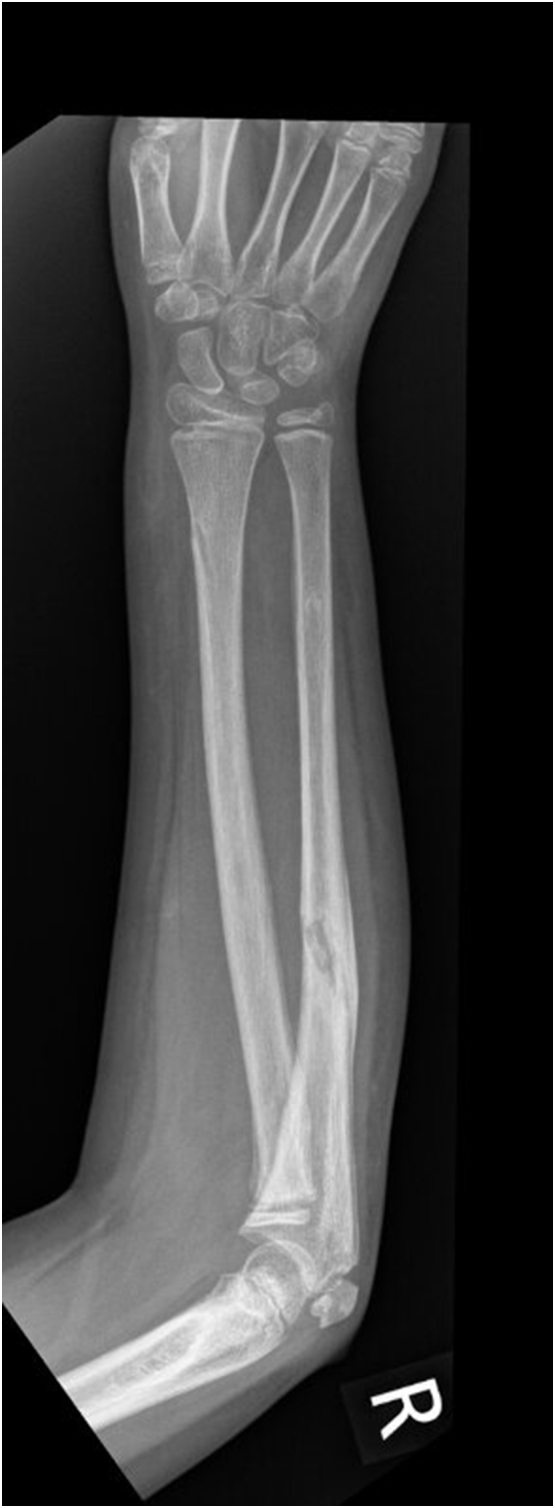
Follow-up x-rays one week after removal of hardware.
